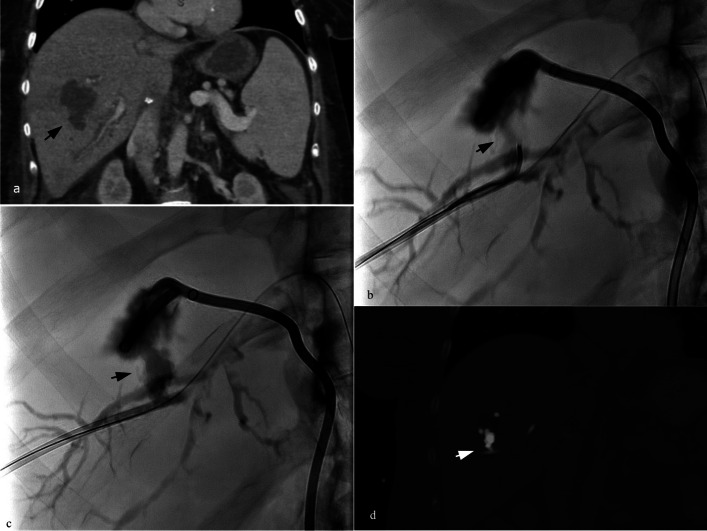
a Contrast enhanced CT abdomen demonstrate a 5.1 cm × 3.6 cm biloma seen within right hepatic lobe. b Catheterization of abnormal bile duct-biloma connection. c Post embolization image with Obsidio embolic within connection. d Coronal view of CT abdomen demonstrating post embolization changes
